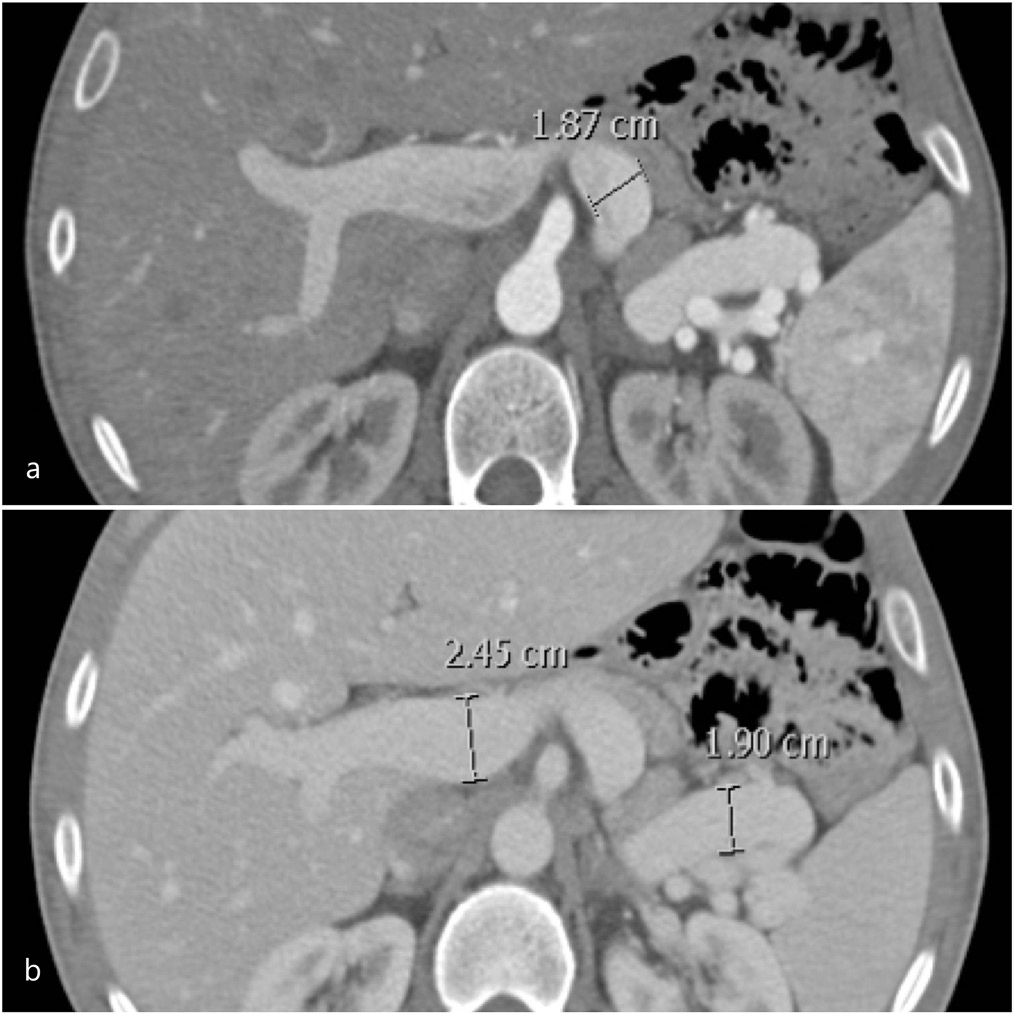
(a, b). Enhanced CT abdomen in portal venous phase showed a fusiform aneurysmal dilatation of the portal vein (2.45 cm) and splenic vein (1.87 cm). The splenic artery is also prominent (1.9 cm).
